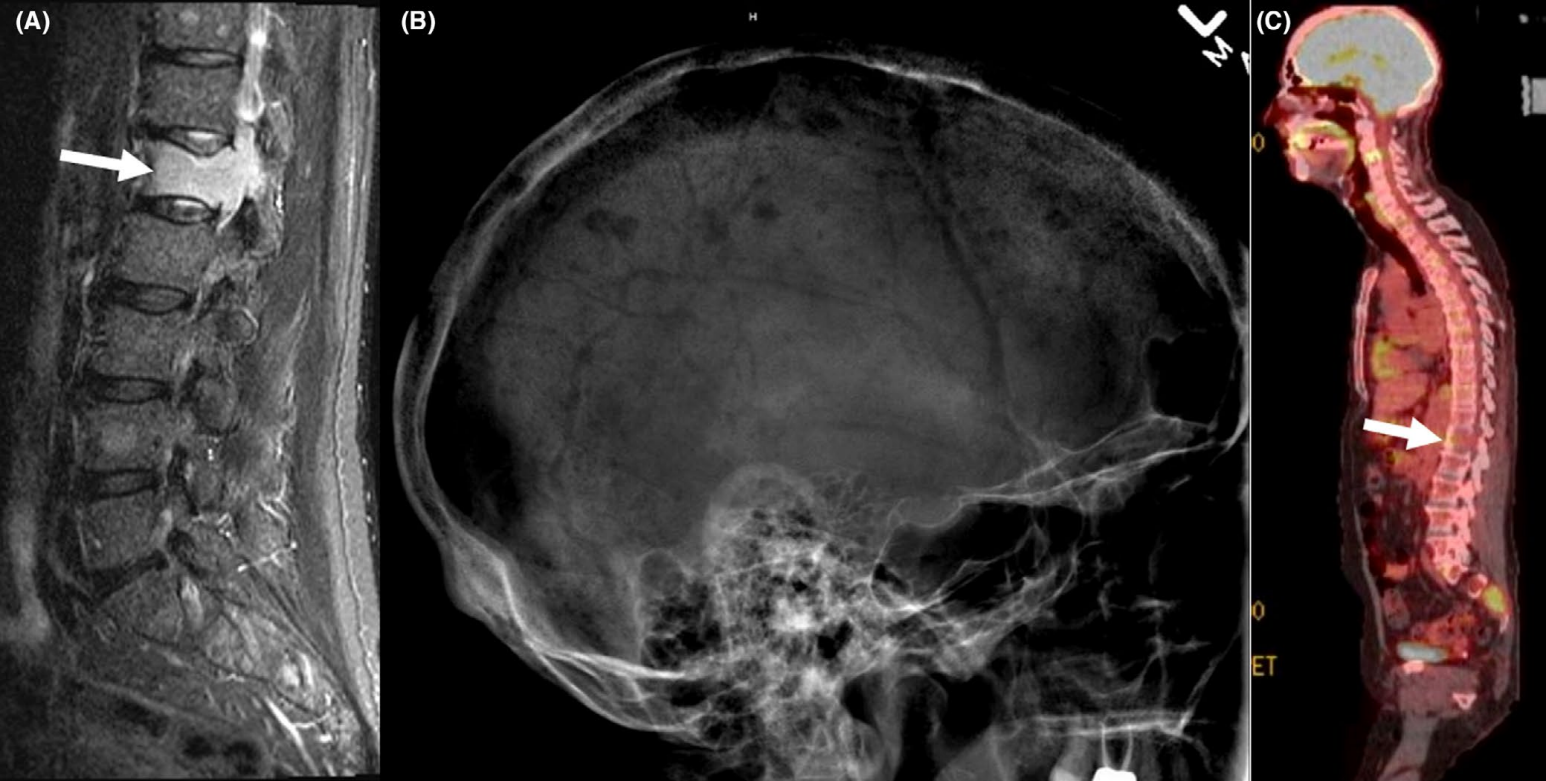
A, Sagittal fat suppressed T2 weighted MR image of the lumbar spine shows a pathologic fracture of the L1 vertebral body with bone marrow edema (arrow). Additional lesions are present in the T11 and L4 vertebral bodies. B, Lateral radiograph of the cranium shows multiple well defined “punched out” lytic lesions. C, Sagittal fusion image from PET/CT shows multiple lytic lesions in the spine with variable FDG uptake. A large lytic lesion is noted in the L1 vertebral body (arrow)
